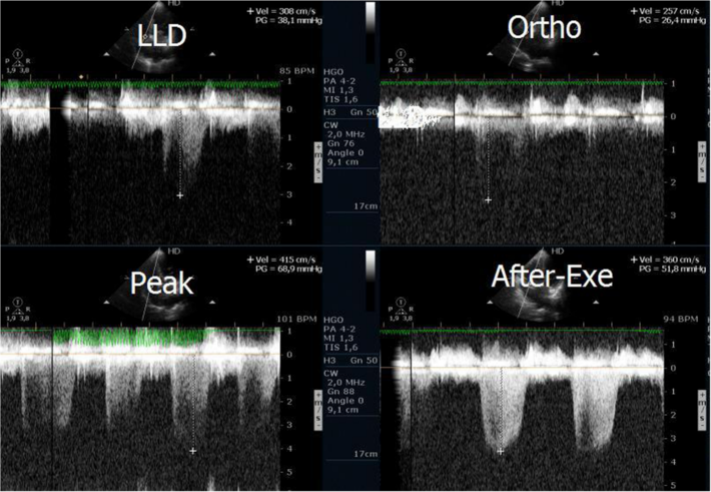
Right ventricular/ right atrium gradient, evaluated with CW Doppler, at different stages of the study in one patient with mitral stenosis.
